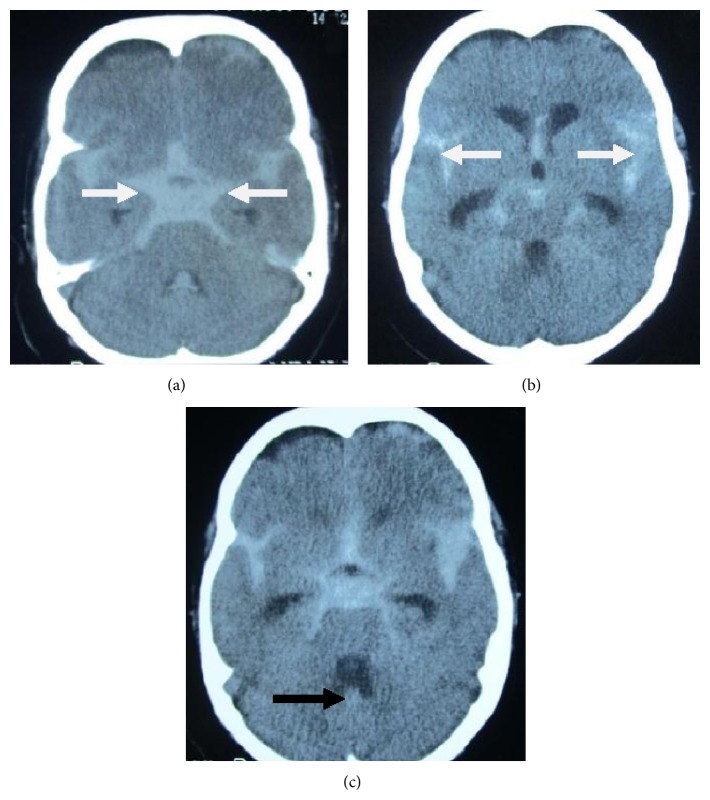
Second CT scan brain without contrast showing clearly visible subarachnoid bleed. (a) Classical star shaped hyperdensity seen filling the subarachnoid space most apparently around the circle of Willis (white arrows); (b) hyperattenuating signal in bilateral sylvian fissures (white arrows); (c) trickle of blood seen extending into the ventricular system (black arrow) (also visible in (a)).
